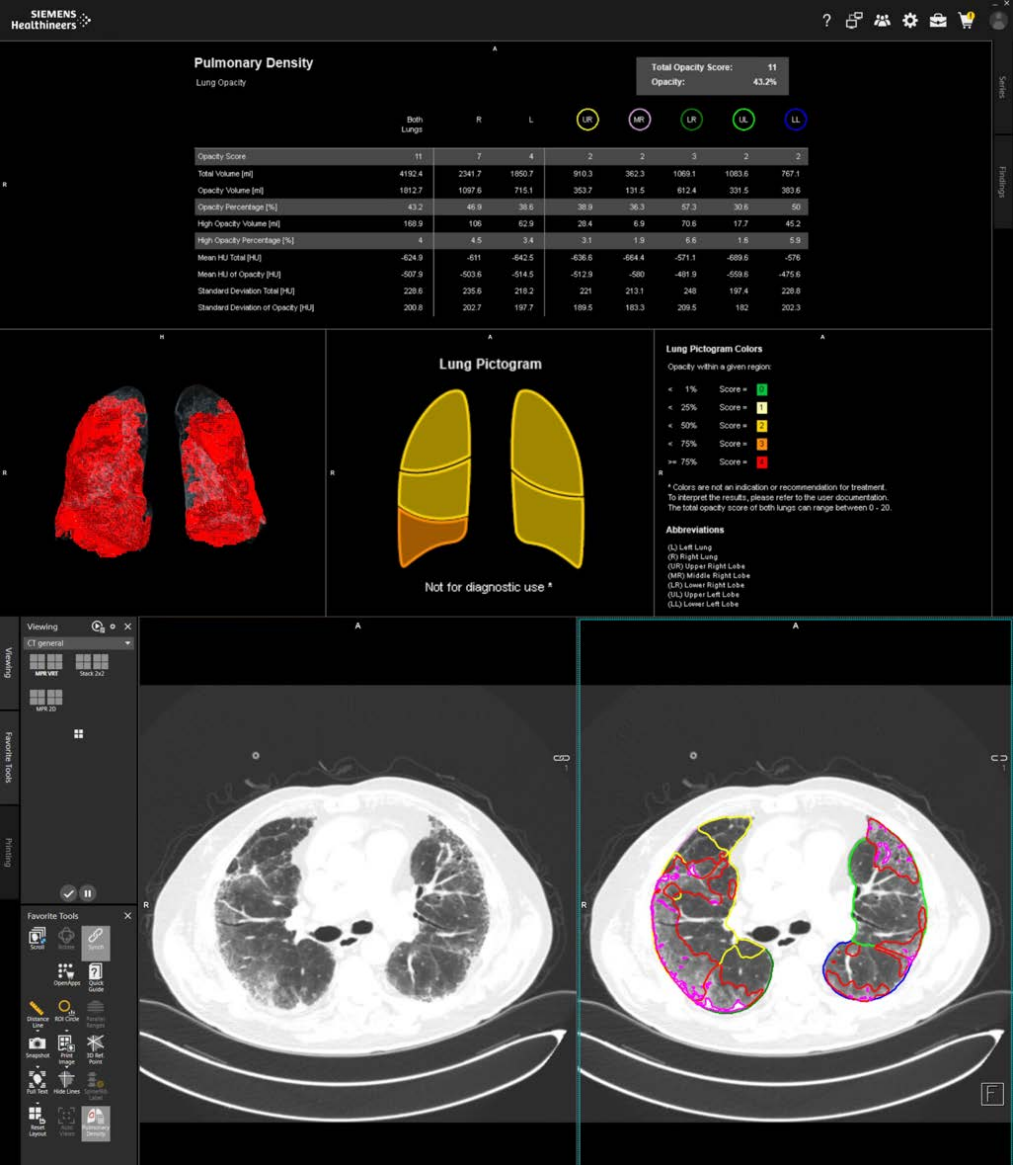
Output of the Siemens CT Pulmonary Density module
